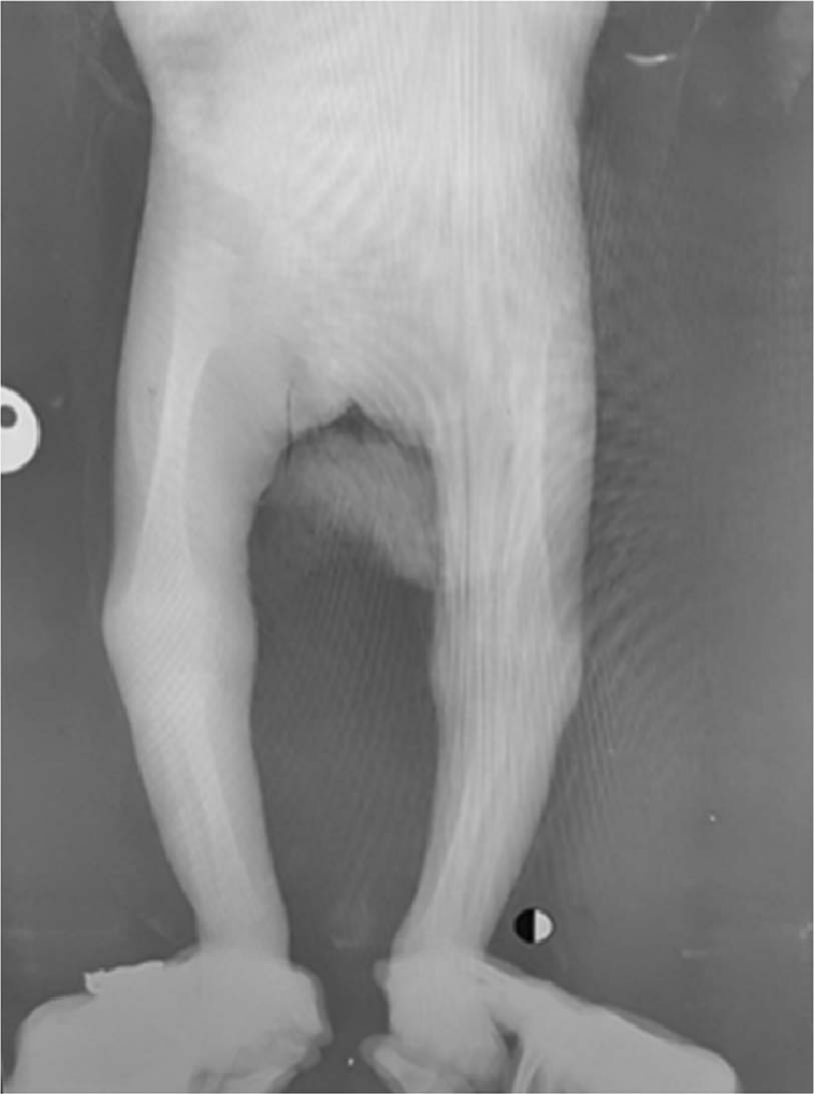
Normal X-rays of the lower limbs.
